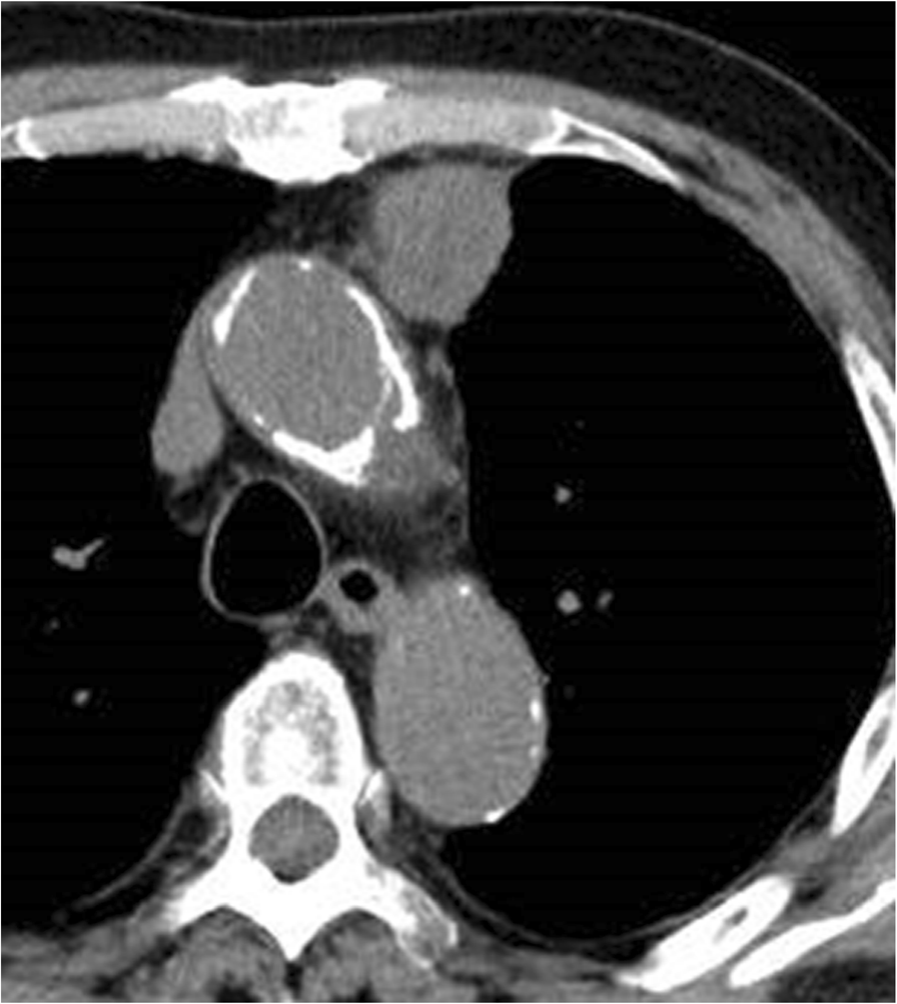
CT findings. CT shows a 31 mm × 28 mm, rough-shaped mass in the anterior mediastinum. It is located mainly in the mediastinum, not in the lung
